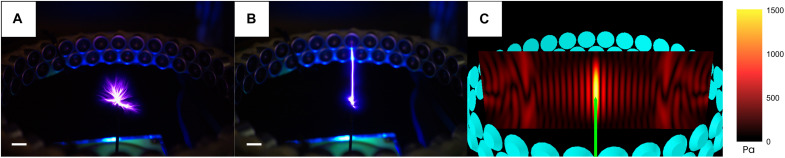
Ultrasonic field guiding electric plasma. (A) Plasma spark without the ultrasonic field applied. (B) Plasma spark with the ultrasonic field. (C) Amplitude of the acoustic field (electrode in green). Scale bars, 1 cm.
